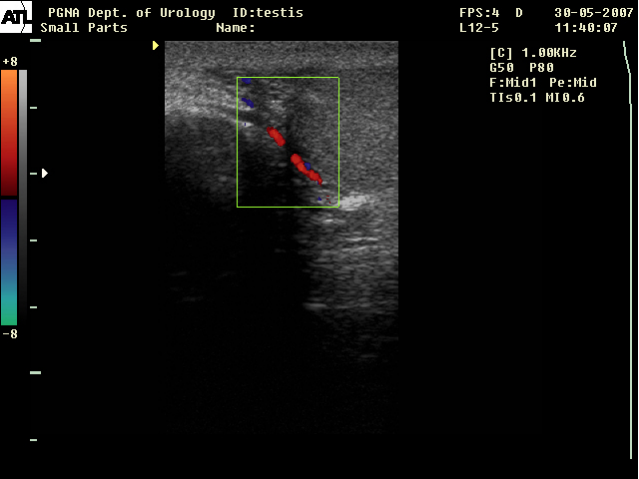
Color Doppler Ultrasound of the right hemiscrotum showing normal testicular parenchyma and blood flow.
